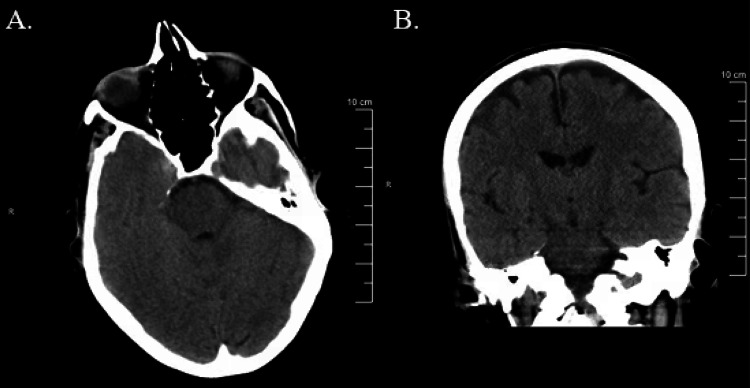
Negative non-contrast CT head axial and coronal view, respectively, at the time of initial presentation in the ED. No focal neurological deficits were present at that time.
